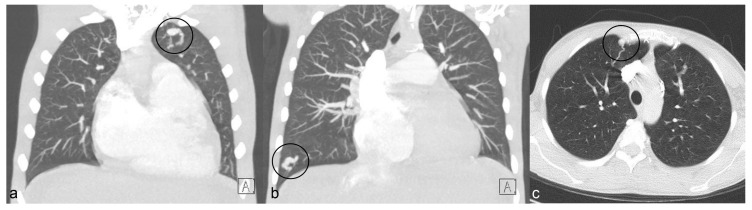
Contrast-enhanced chest CT. Coronal MIP (a,b) and axial (c) images show peripheral AVMs, seen as non-calcified nodules with a feeding arterial vessel (within black circle in b). MIP: Maximum Intensity Projection.
